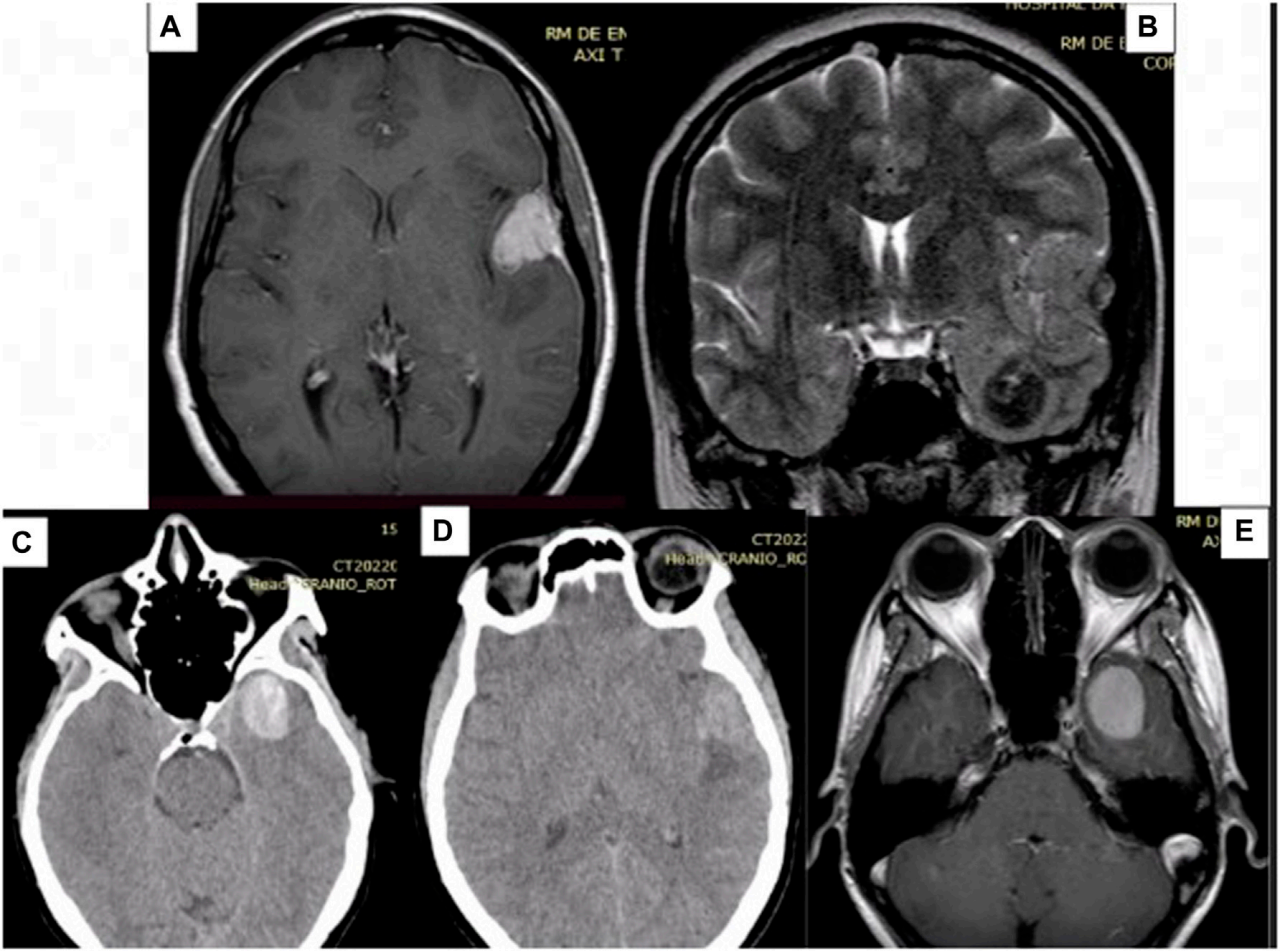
Preoperative MRI images showing two lesions, one on the floor of the middle fossa and the other in the pterygium region, both on the left. (A) Contrast-enhanced T1-weighted MRI of the pterional lesion, which appears hyper-intense and with homogeneous contrast uptake. (B) Coronal T2 MRI showing the two lesions. (C) Axial CT scan, without contrast, showing that the deeper lesion is hyper-dense in relation to the parenchyma. (D) Axial CT scan without contrast. (E) Contrast-enhanced T1-weighted MRI of the deepest lesion, which appears hyper-intense and with homogeneous contrast uptake. MRI: Magnetic Resonance Imaging. CT: Computed Tomography.
